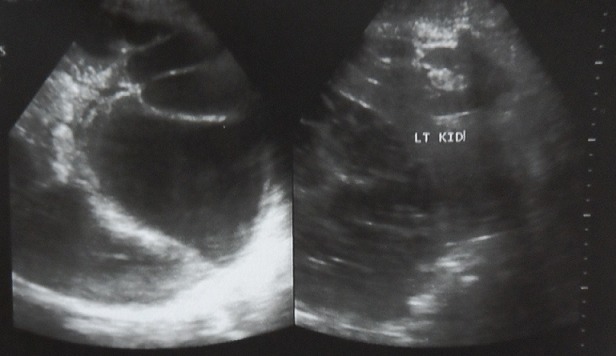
Ultrasound images of the liver shows a large dominant ‘mother cyst’ containing multiple septa between peripherally placed daughter cysts. This cyst-within-cyst appearance is also referred to as the ‘Spoke wheel sign’ or the ‘Honey comb sign’ and is typical of Hydatid Disease
